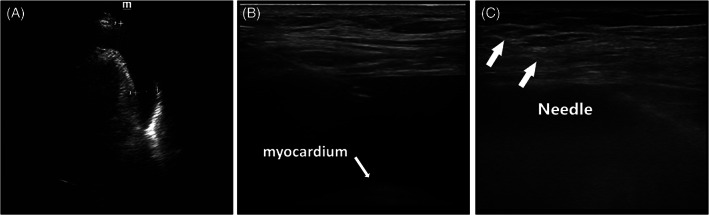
Representation of large symptomatic MPE in a 72‐year‐old man with a history of lung cancer. (A) Conventional echocardiogram performed before the procedure showed a large pericardial effusion. The maximal anterior depth was 29 mm. (B) The apical view was obtained with the high frequency probe and the depth adjustment was 4 cm. The needle is continuously visualized by a high frequency ultrasound while entering the pericardial space. (C) After the needle penetrated through the pericardial space, a j‐tipped guidewire was gently advanced through the needle
